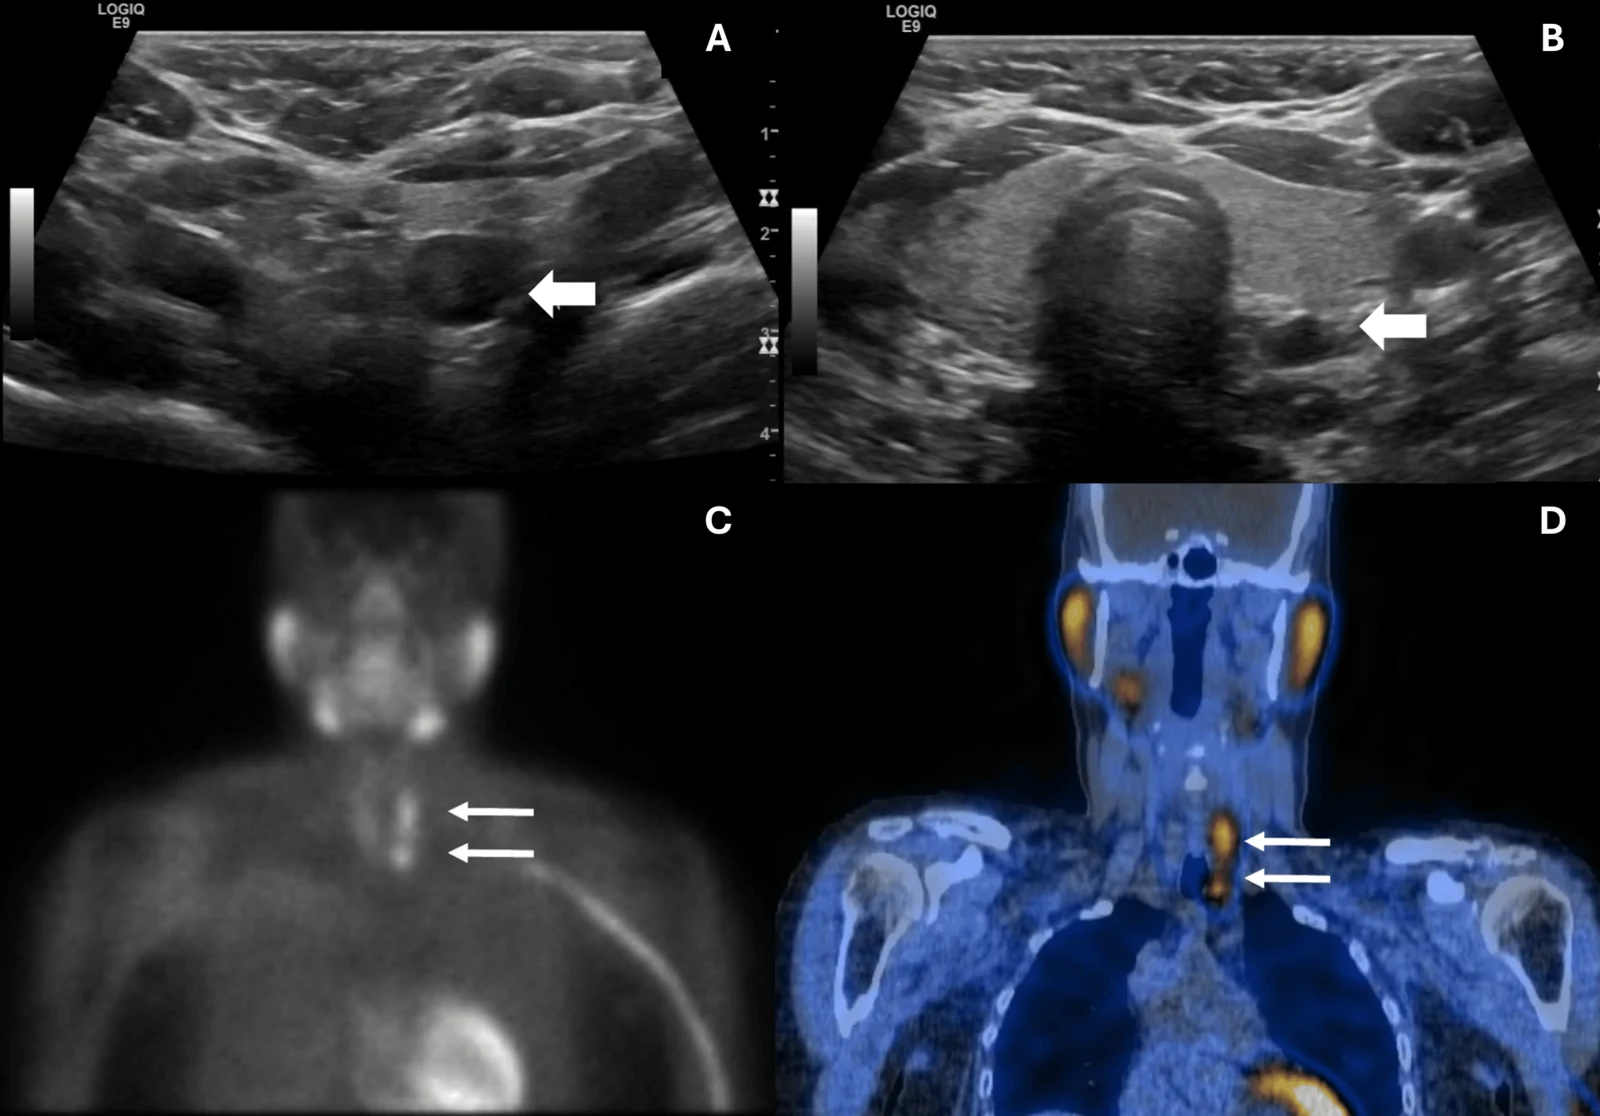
Preoperative imaging demonstrating left-sided double parathyroid adenomas (A) Transverse neck ultrasound demonstrating a left inferior parathyroid adenoma (arrow). (B) Transverse neck ultrasound demonstrating a left superior parathyroid adenoma (arrow). (C) Technetium-99m sestamibi (Tc-99m MIBI) scintigraphy, grayscale image, demonstrating radiotracer uptake corresponding to the left-sided double parathyroid adenomas (arrow). (D) Color-enhanced Tc-99m MIBI scintigraphy demonstrating radiotracer uptake corresponding to the left-sided double parathyroid adenomas (arrow).
